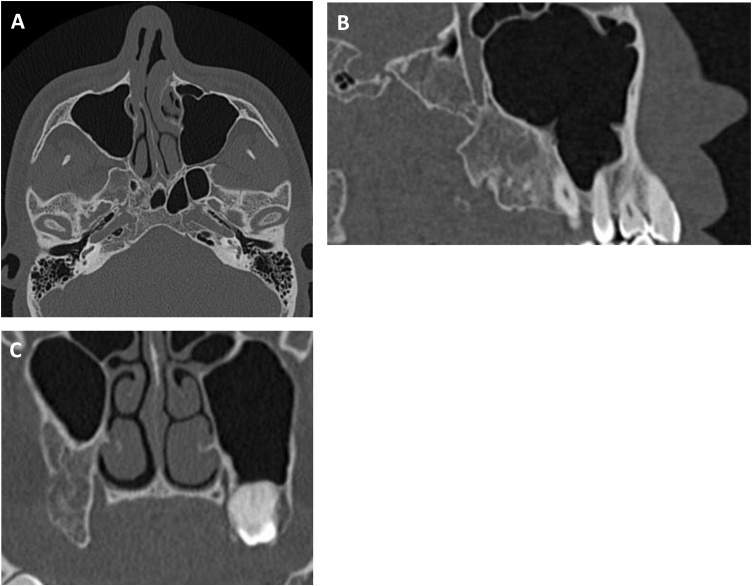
Follow-up CT scans show no recurrent lesion after a secondary cystectomy. (A) Axial scan. (B) Sagittal scan. (C) Coronal scan.
